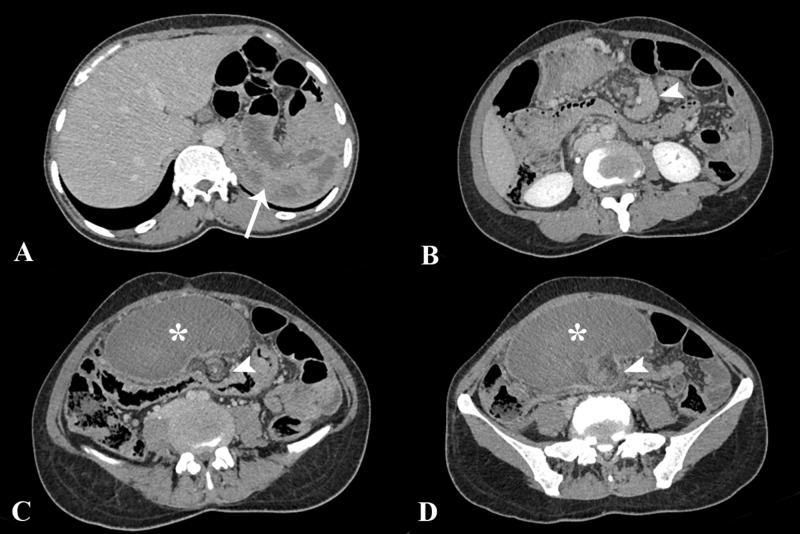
Contrast-enhanced computed tomography images. Axial sections showing absence of spleen in its normal position in the left upper quadrant (arrow). An enlarged spleen (asterisk) was found located in the lower abdomen, reaching up to the pelvis. Low attenuation of the splenic parenchyma was noted with elongated and twisted vascular pedicle (arrowheads). The pancreatic tail was also involved in the twisting of the pedicle as seen in B (arrowhead).
